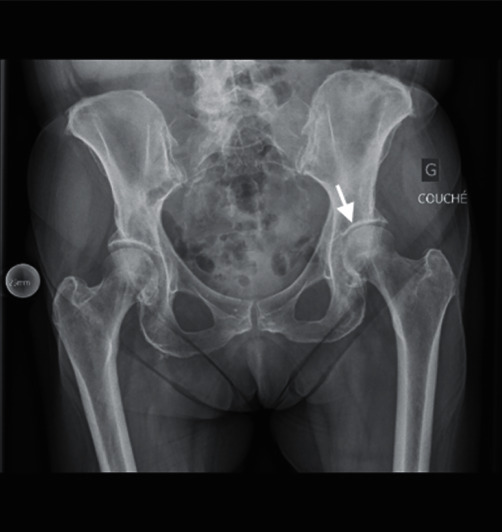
Pelvic anteroposterior view 6 weeks after initial injury. Compared with earlier radiographs, a superior joint space narrowing can be seen, as well as osteosclerosis of the acetabulum (arrow).
